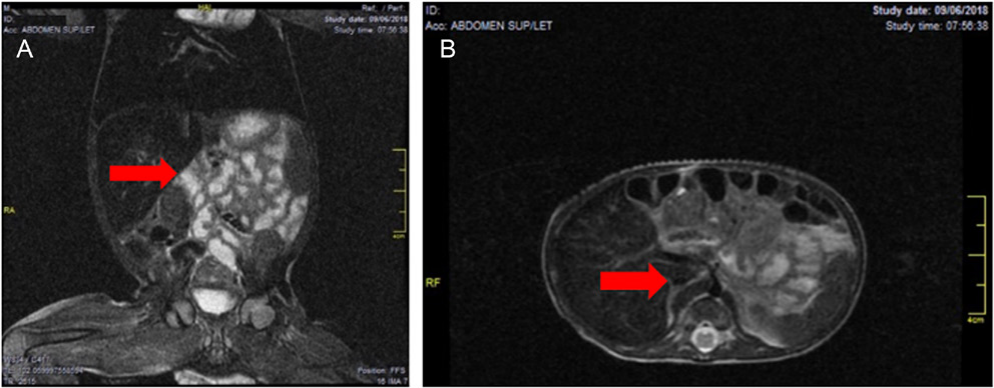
Nuclear magnetic resonance images of the abdomen of the first male patient case: (A) coronal section and (B) sagittal section; the arrow indicates the site where the pancreas should be visualized.
